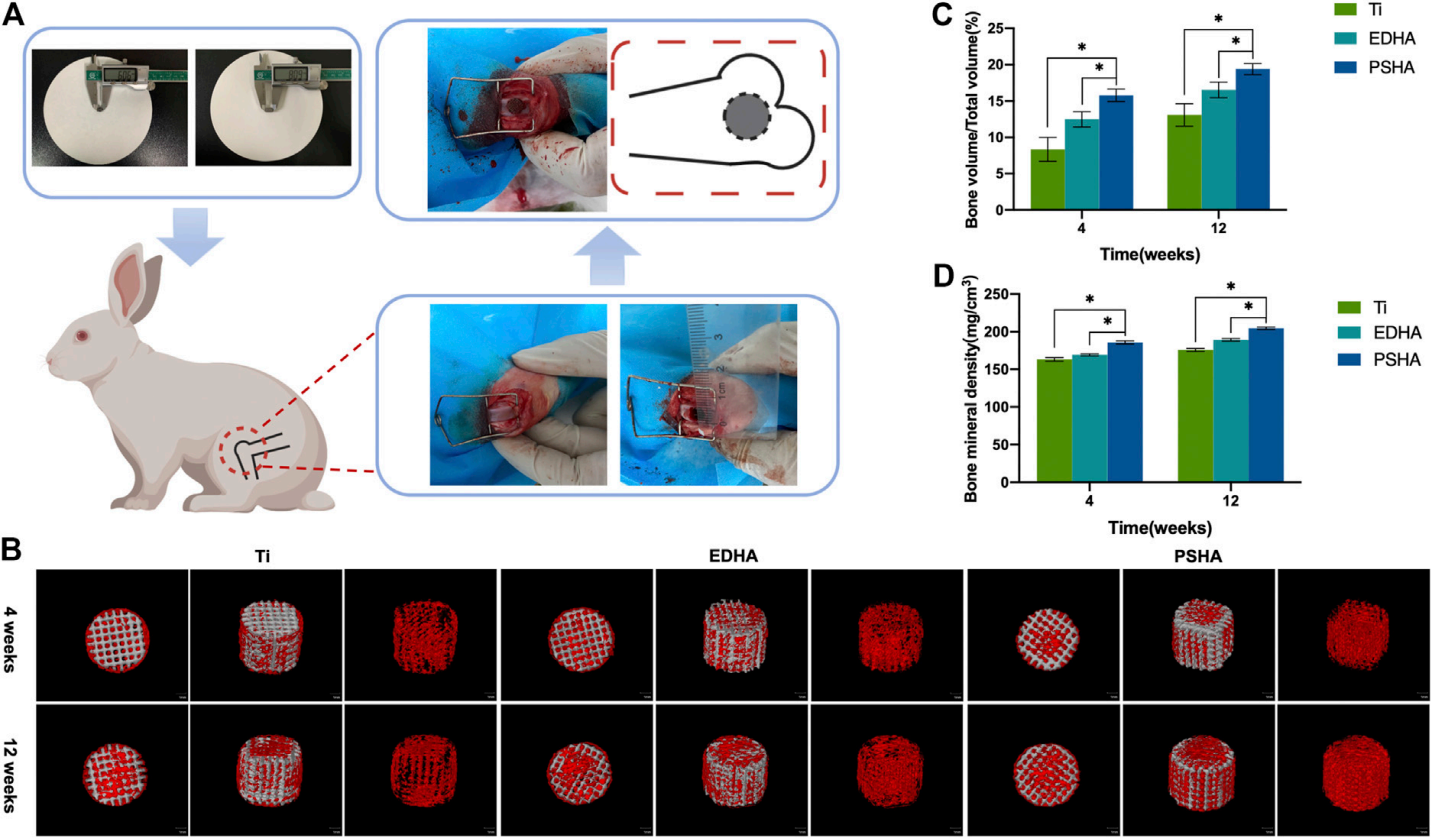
(A) Construction of bone defect and implantation of designed scaffolds. (B) 3D reconstruction of bone tissues through micro-computed tomography (CT) analysis at 4 and 12 weeks (red: new bones; grey: scaffolds). Quantitative analysis of (C) bone volume/total volume (BV/TV) and (D) bone mineral density (BMD).
